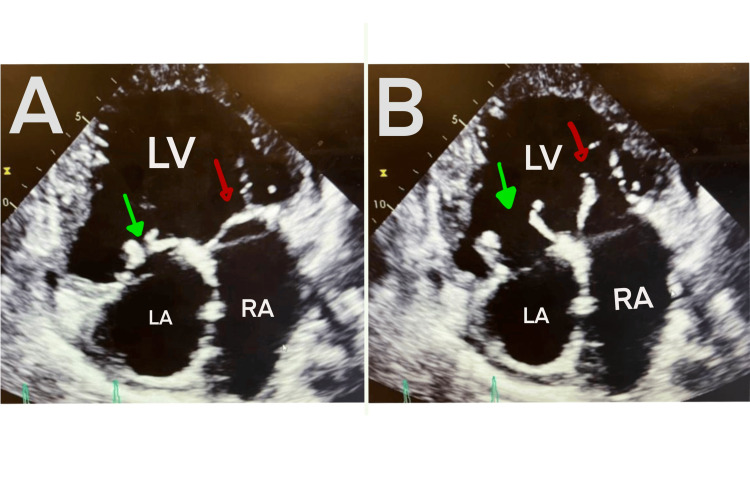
Apical 2D echocardiogram of the patient displaying a single ventricle Panel A: with valves closed; Panel B: with valves open; Red arrow: tricuspid valve; Green arrow: mitral valve; LA: left atrium; RA: right atrium; LV: left ventricle
